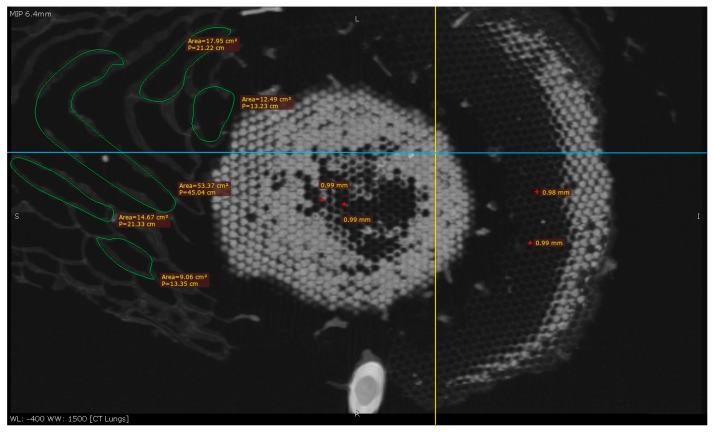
Asian hornet nest measurement using CT scan.
